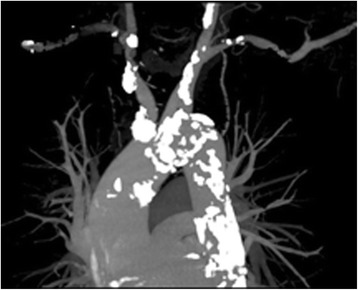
Preoperative computed tomography image showing severe calcification of the aortic arch and supra-aortic trunks
